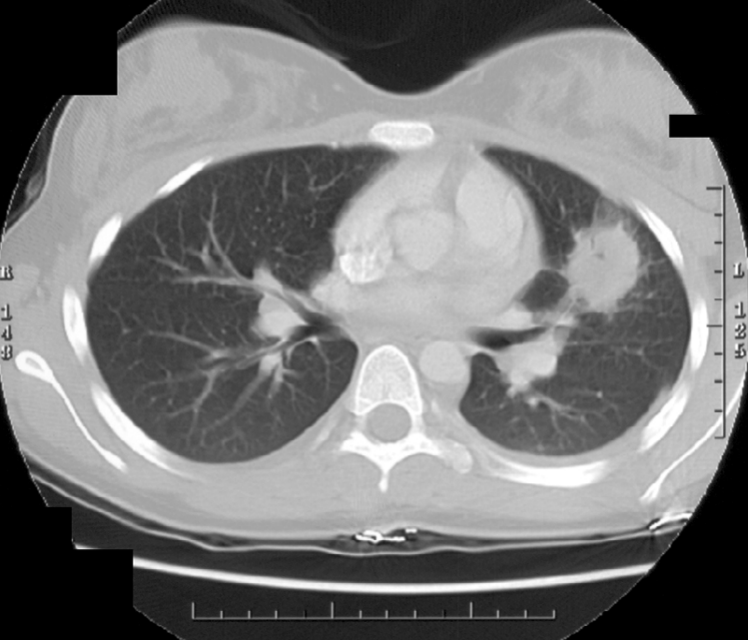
Chest CT scan showing a well-circumscribed homogeneous pulmonary mass (4 cm) within the lingual of the left lung in a newly diagnosed child with ulcerative colitis.
